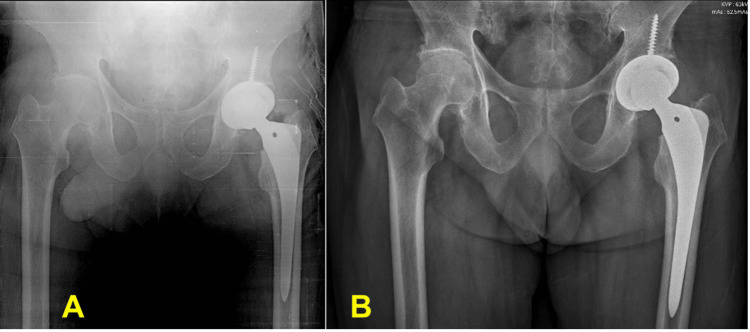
(A) Postoperative X-ray immediately after surgery; (B) Follow-up X-ray, with 12 weeks of progression.
